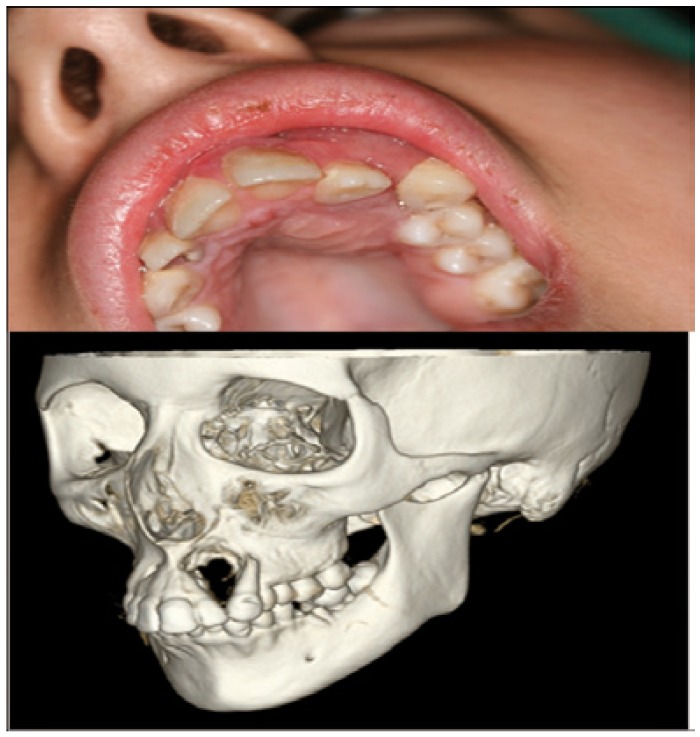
a Initial clinical view of the lesion. b View of the defect created by the lesion in 3D reconstruction CT-scan.
